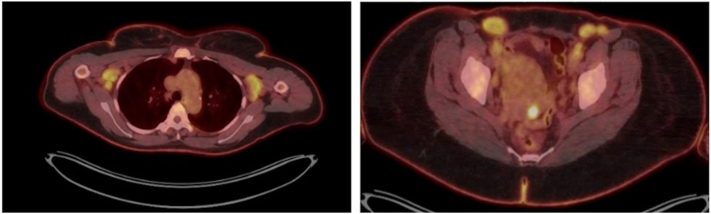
Initial whole-body positron emission tomography/computed tomography showing multiple fluorodeoxyglucose-avid enlarged bilateral axillary *(left)* and inguinal *(right)* lymph nodes, suggestive of lymphomatous involvement. The dominant right inguinal lymph node, measuring 3.4 × 2.3 cm, was subsequently biopsied, showing florid lymphomatous involvement (N3).
